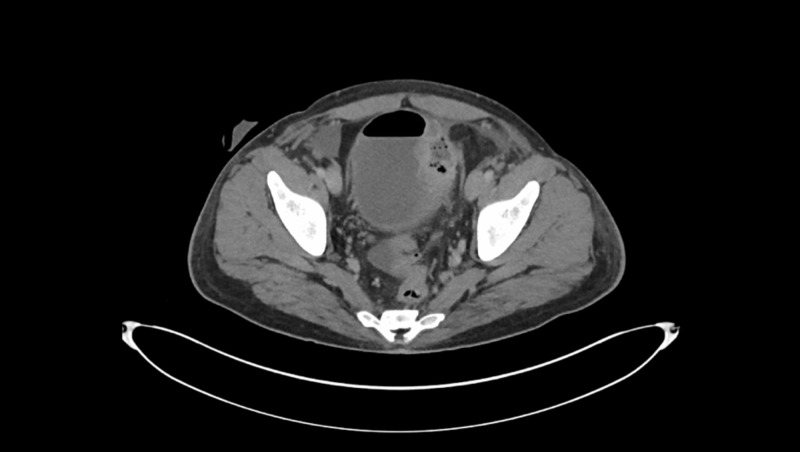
CT abdomen/pelvis done in October 2019. Depicting left pelvic collection possible sigmoid abscess/communication of colovesical fistula.
